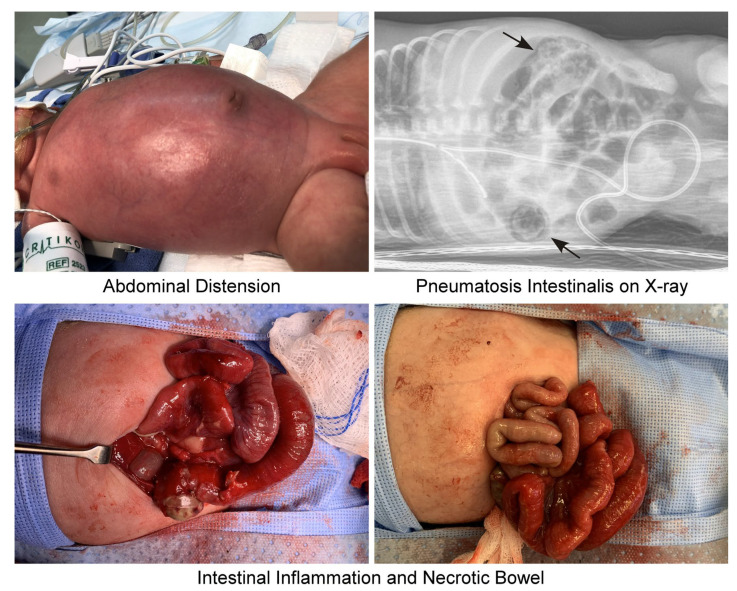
Clinical symptoms of NEC. NEC presents with a variety of clinical and radiological signs, requiring the Bell’s staging criteria to guide treatment and management of the disease. Earlier findings include abdominal distension (top left) and bloody stool. On X-ray, NEC presents with pneumatosis intestinalis or gas in the intestinal wall (top right, black arrows). Worsening of the disease, during which surgical intervention would be required, involves perforated intestinal damage and necrotic bowel tissue (bottom). Images were taken at Stony Brook Medicine by Helen Hsieh (author) and de-identified.
